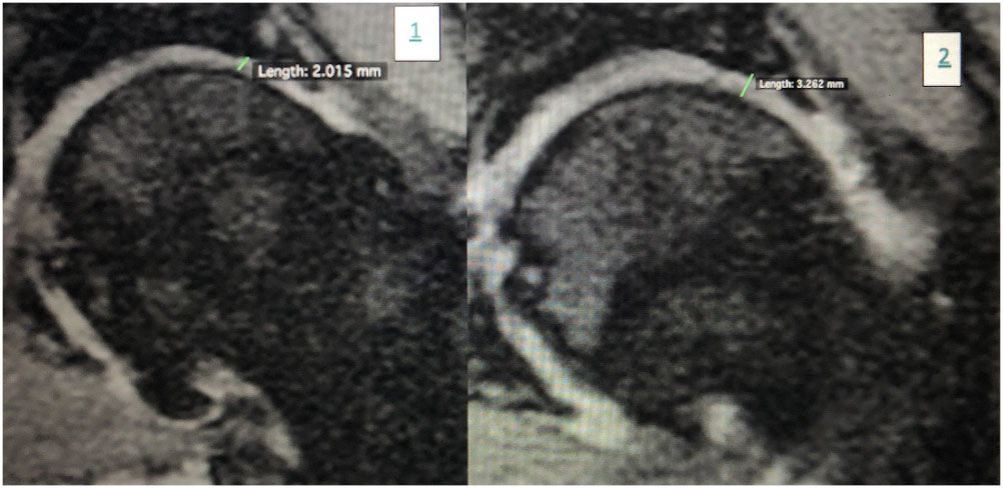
MRI (Siemens 3,0 T—t1_tirm_cor) of a 31 years old man. (1) Pre-operative image, with the chondral defect and chondrolysis in the acetabulum visible. Joint space 2 mm (2) MRI image of the same patient 1-year post-operatively with ChondroFiller gel. Joint space 3.2 mm.
